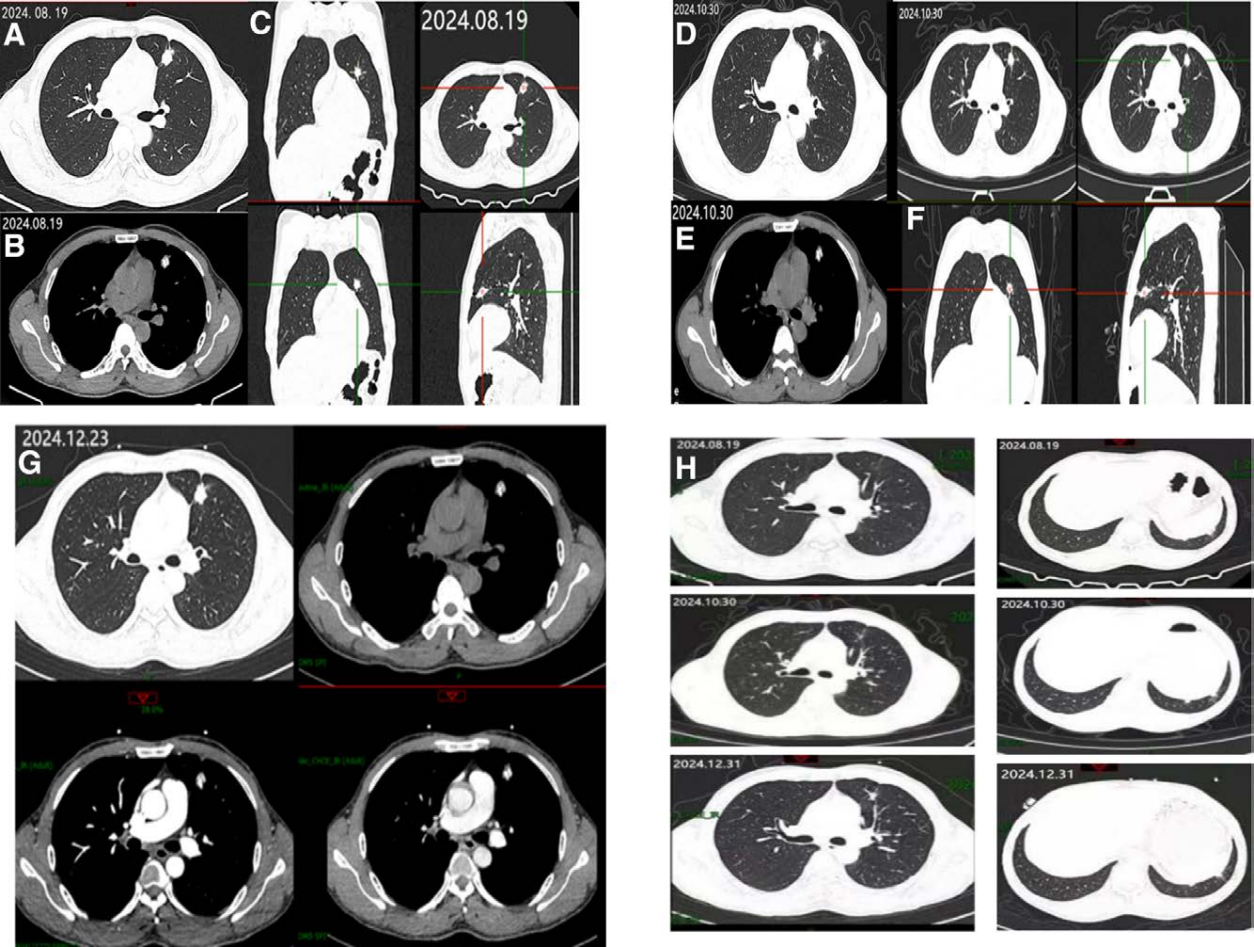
August 19, 2024 CT, (A) lung window, (B) mediastinal window, (C) lung window MPR. October 30, 2024 CT, (D) lung window, (E) mediastinal window, (F) lung window MPR. December 23, 2024 CT, (G) changes in pulmonary window, mediastinal window, arterial phase, delayed phase, (H) changes in oblique fissure nodules in patients. CT = computed tomography, MPR = multi-planar reformation.
